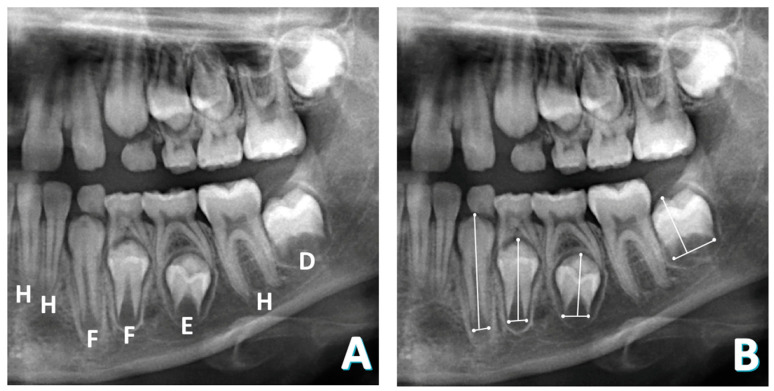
Dental age determination methods in panoramic radiograph. (A) Demirjian’s method; (B) Cameriere’s method.
